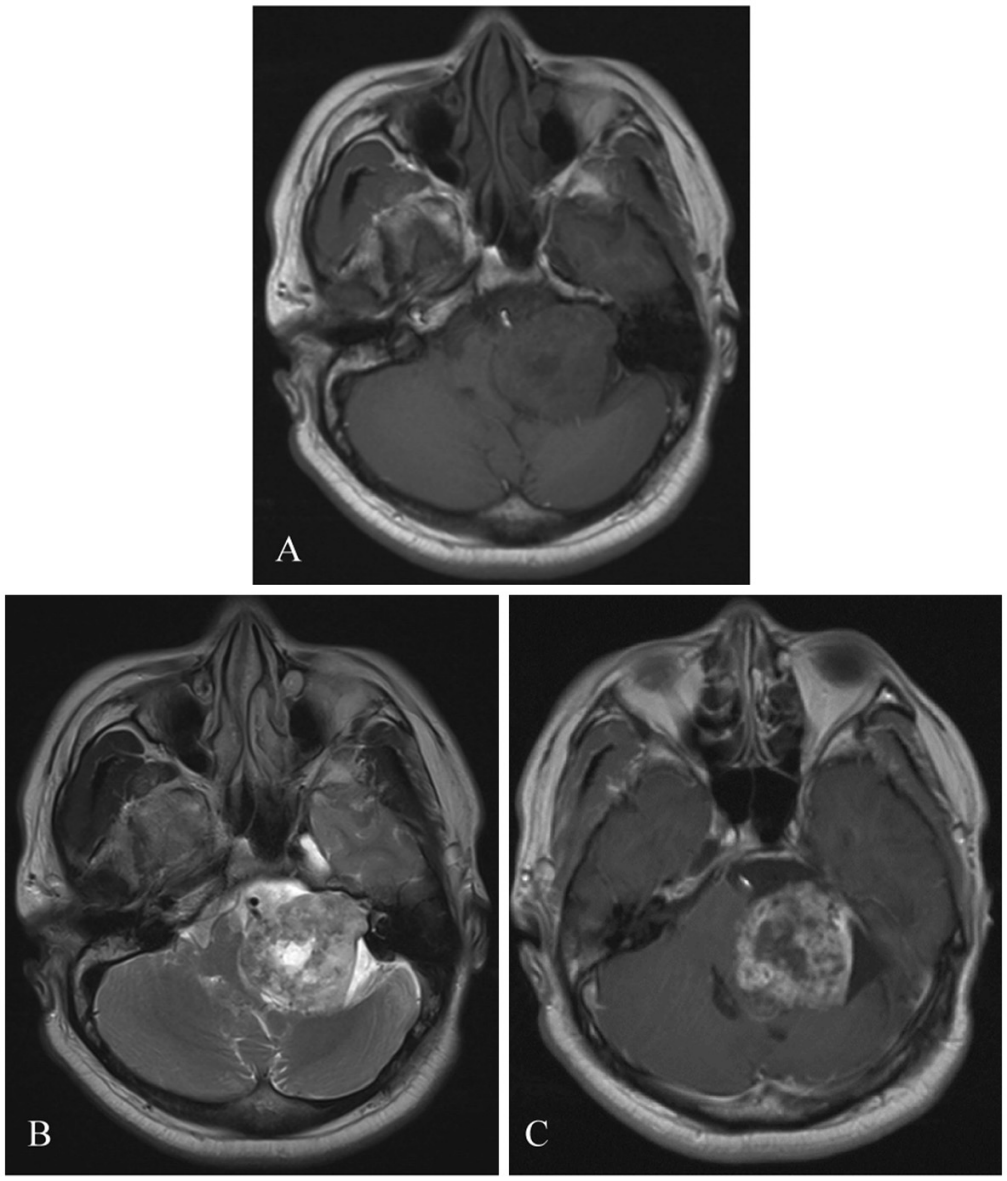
Preoperative MRI showing a round mass with a size of 5.42 × 4.27 × 5.35 cm in the left CPA region. (A) Hypointensity on the T1-weighted imaging (T1WI). (B) Uneven hyperintensity on the T2-weighted imaging (T2WI). (C) Heterogeneous enhancement after contrast injection. The left cerebellum, the fourth ventricle, and the brain stem were compressed.
